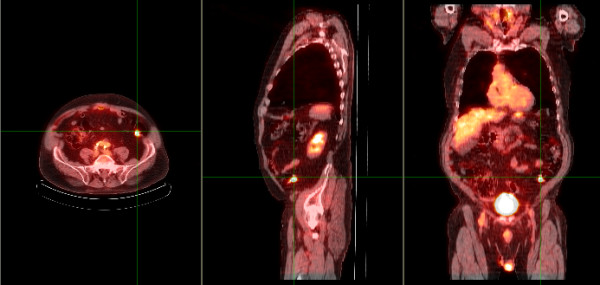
Fused coronal, sagittal and transaxial FDG PET/CT images in an 84 year old patient (Patient 11). The cross-hairs localize intense uptake in the left colon.
